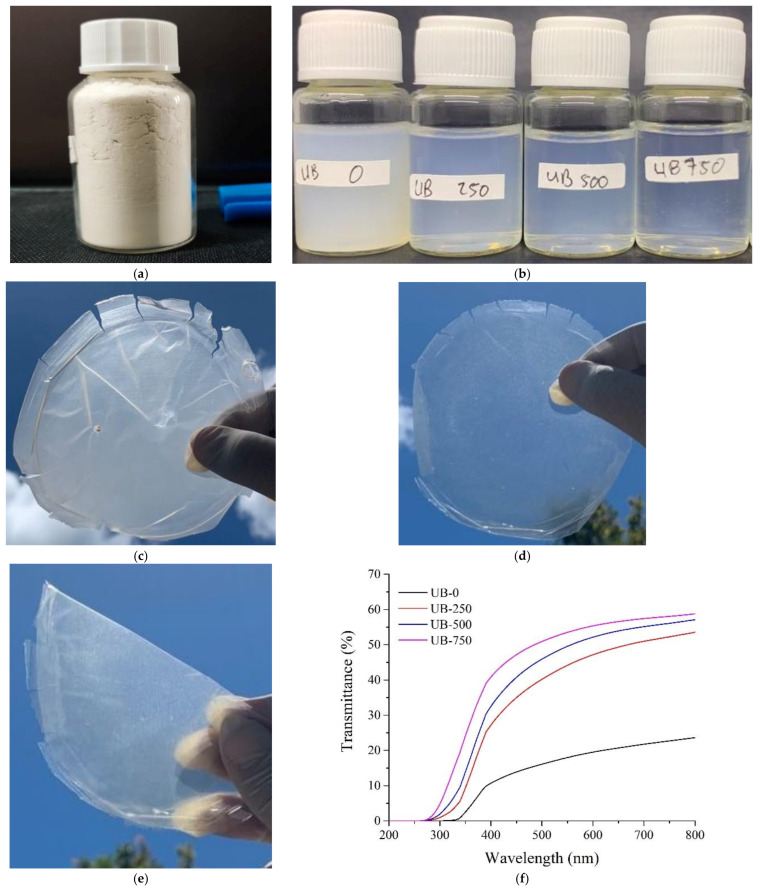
(a) BC powder, (b) appearance of treated BC suspension from various ultrasound energy, (c) translucent UB-0 film, (d) transparent UB-750 film, (e) bendable UB-750 film, (f) transmittance value of films.
